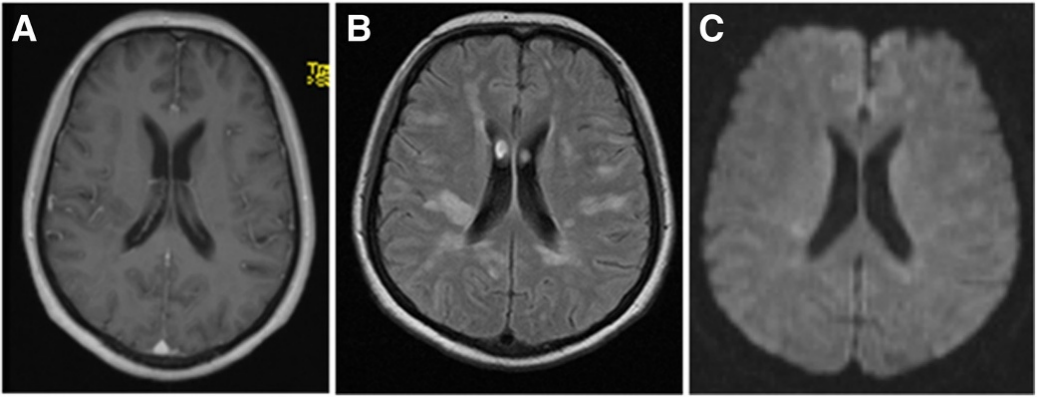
MRI-scan two days after symptom onset. Emerging white matter lesions with slight restricted diffusion but without Gadolinium (Gd) enhancement. A) T1 Gd-enhanced, B) T2-FLAIR (fluid attenuated inversion recovery), C) DWI (diffusion-weighted imaging).
